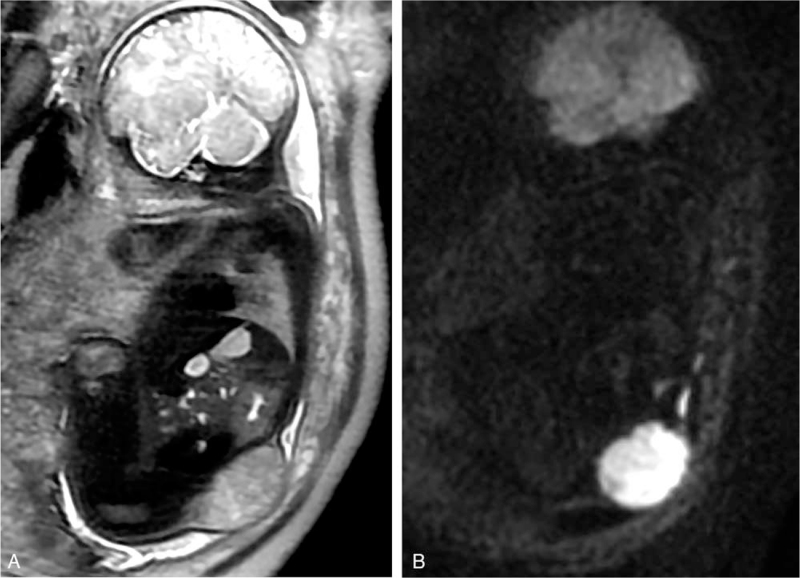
Sagittal T2-weighted imaging at 37 weeks of gestation showed a mixed-signal subcutaneous solid mass located on the left lumbar region (A). On sagittal DWI (b = 800 s/mm^2^), the mass demonstrated significantly high signals (B).
